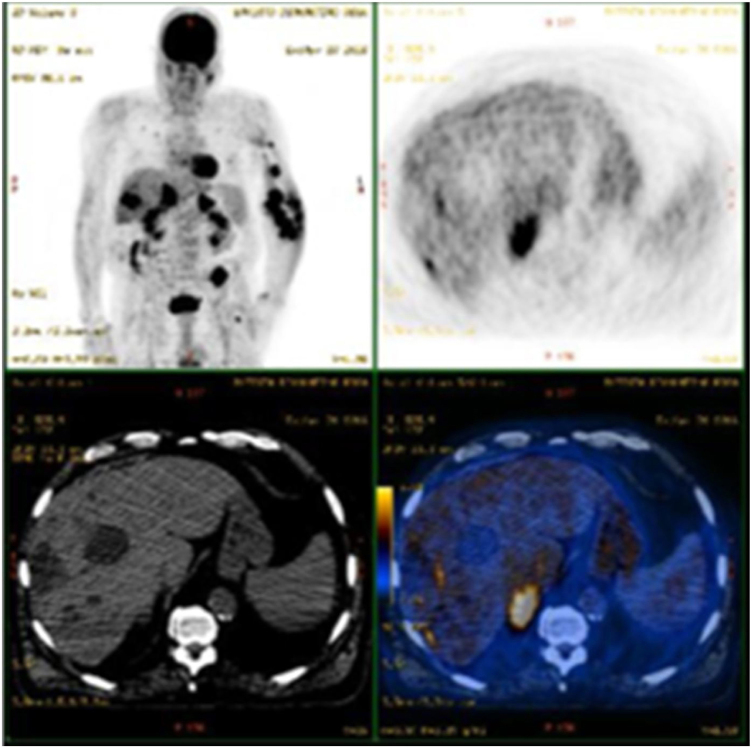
PET-CT scan showing the progression of Kaposi’s Sarcoma with metastatic involvement of the liver in a patient with previously known gastrointestinal and pulmonary involvement. This patient later died despite multiple treatments, including doxorubicin, alfa-interferon, paclitaxel, vinorelbine, and radiotherapy.
